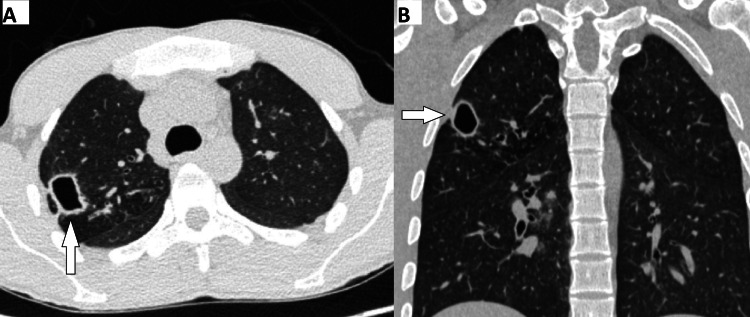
An 18-year-old male patient diagnosed with Mycobacterium tuberculosis following culture from sputum and bronchoalveolar lavage (A) Axial CT image shows a thin-walled cavitary lesion in the right upper lobe of the lung. (B) Coronal CT reformatted image demonstrates the smooth inner margin of the cavity with adjacent ground-glass opacity.
